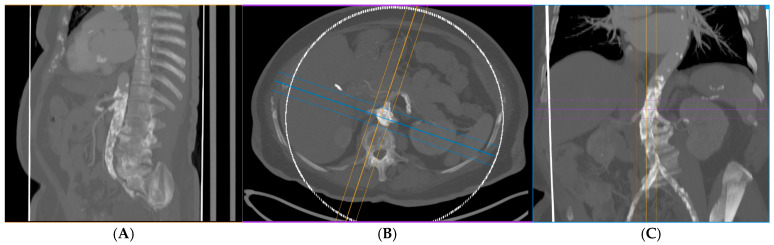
(A) Postoperative computed tomography angiography (CTA) images in sagittal, (B) axial, and (C) coronal planes following endovascular chimney repair for midaortic syndrome. The images demonstrate well-positioned stent grafts with satisfactory patency of the aortic lumen and visceral branch vessels.
